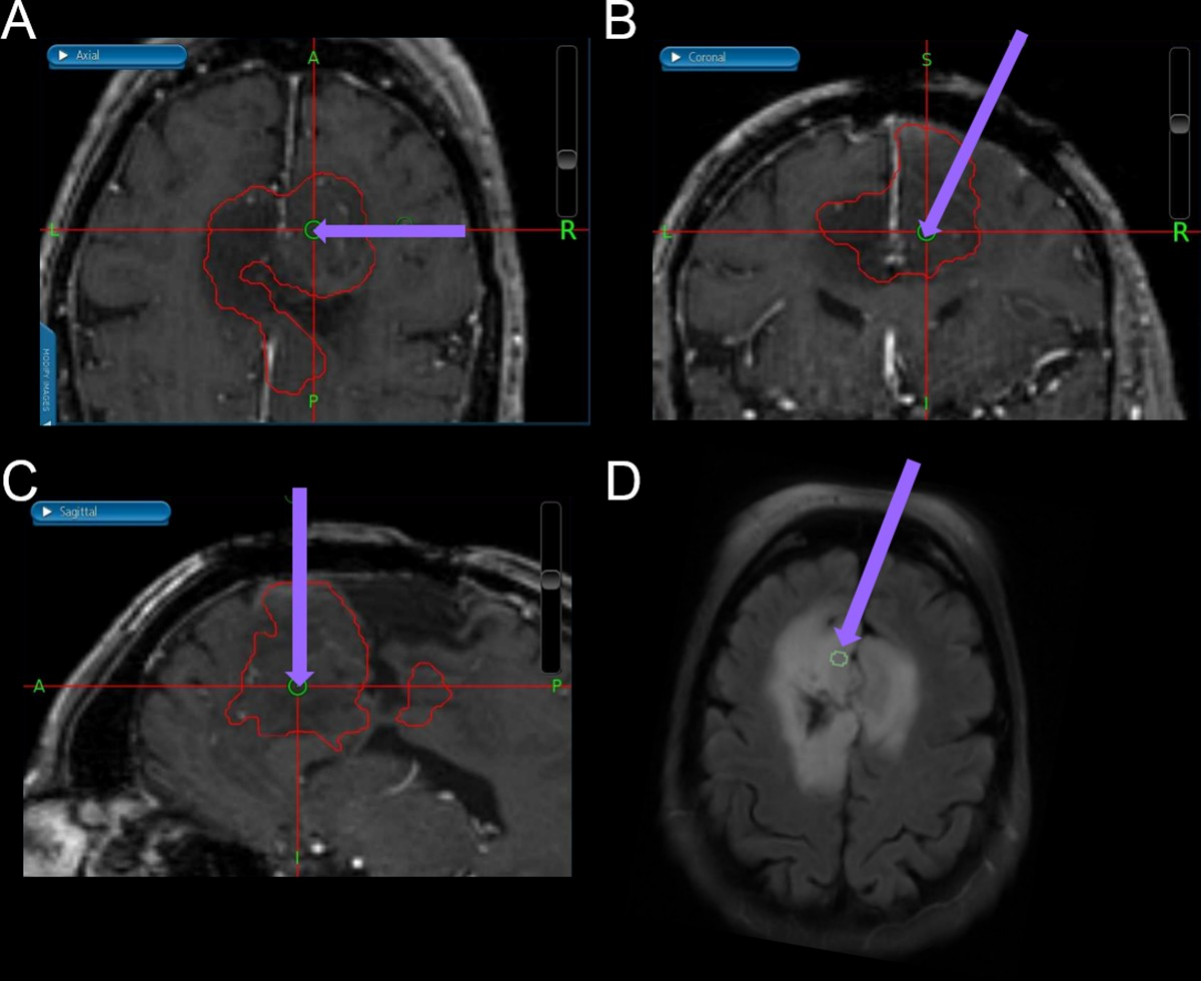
Neuronavigation-guided anatomic images of biopsy tissue sampling sites. Neuronavigation-guided T1-weighted images of the biopsy tissue sampling site at (A) axial, (B) coronal, and (C) sagittal views. (D) The tissue sampling site is labeled on the T2-weighted fluid attentuation inversion recovery image. Purple arrows indicate the passive biopsy needle.
